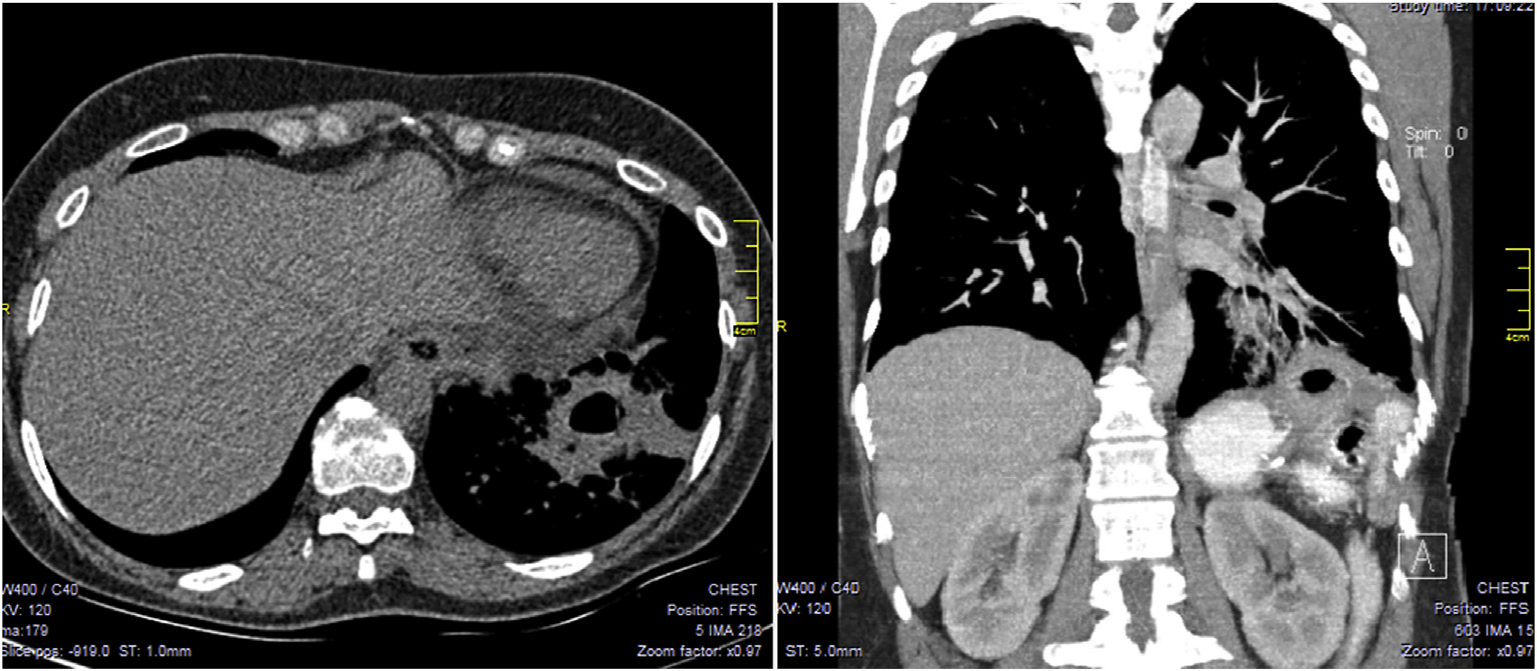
CT-scan of the chest and the upper abdomen showing an evidence of collection in the left sub-diaphragmatic region and consolidation of the left lung zone.
